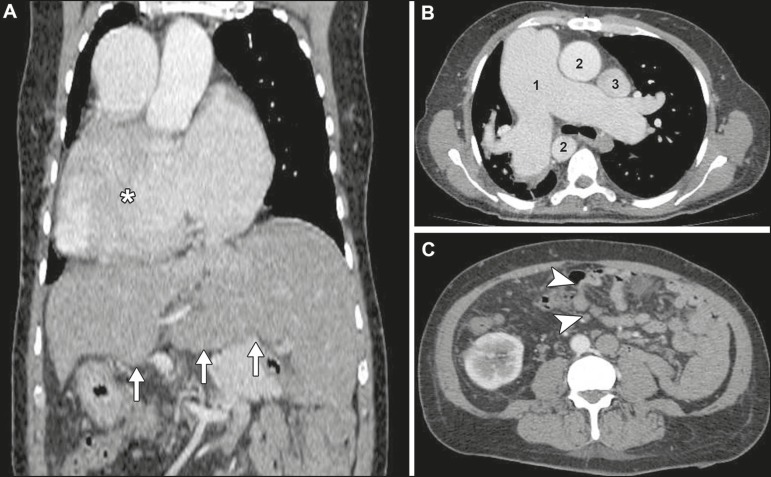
Contrast-enhanced CT scan. A: Coronal thoracoabdominal CT scan showing
